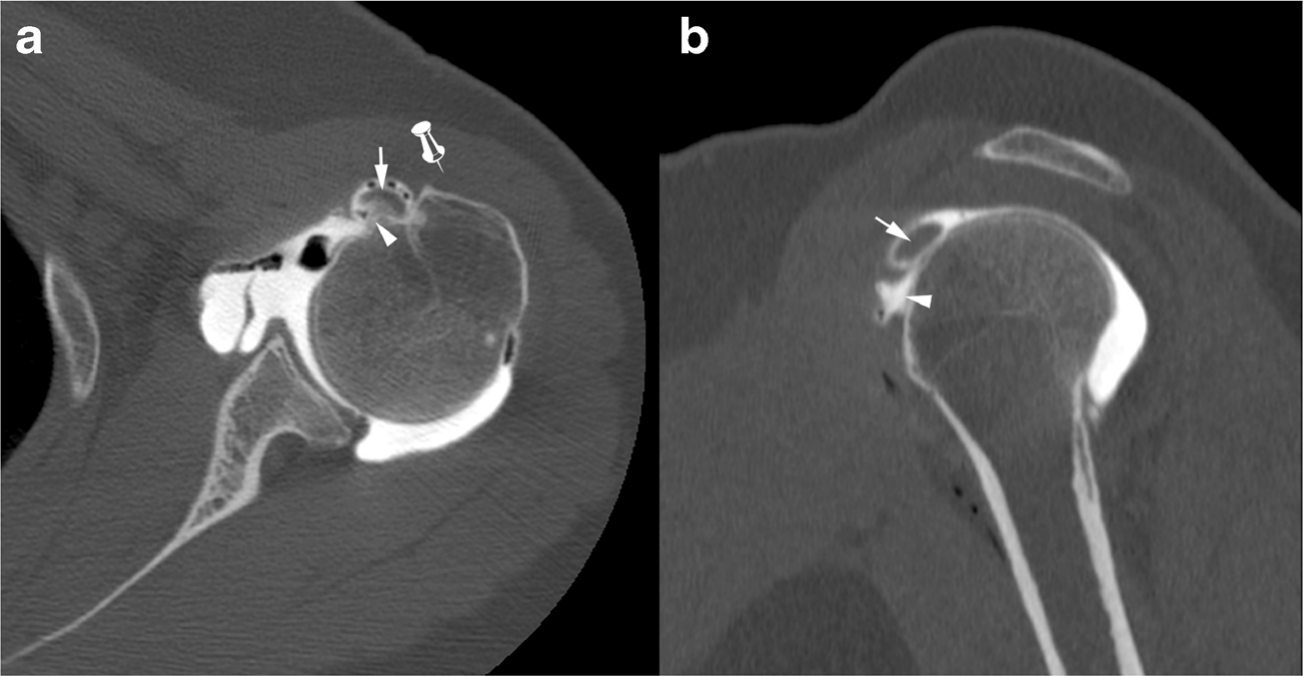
Computed tomography arthrography of the left shoulder with axial (a) and sagittal oblique (b) multiplanar reconstructions in a 38-year-old man, in a post-traumatic context. Isolated complete partial thickness tear of the SSC tendon, located at the articular side of the tendon, involving the upper fibres (arrow head), responsible for subluxation of the LHBT (arrow). Absence of contrast in the SASD bursa (pin) confirms the absence of transfixing or full thickness tear
